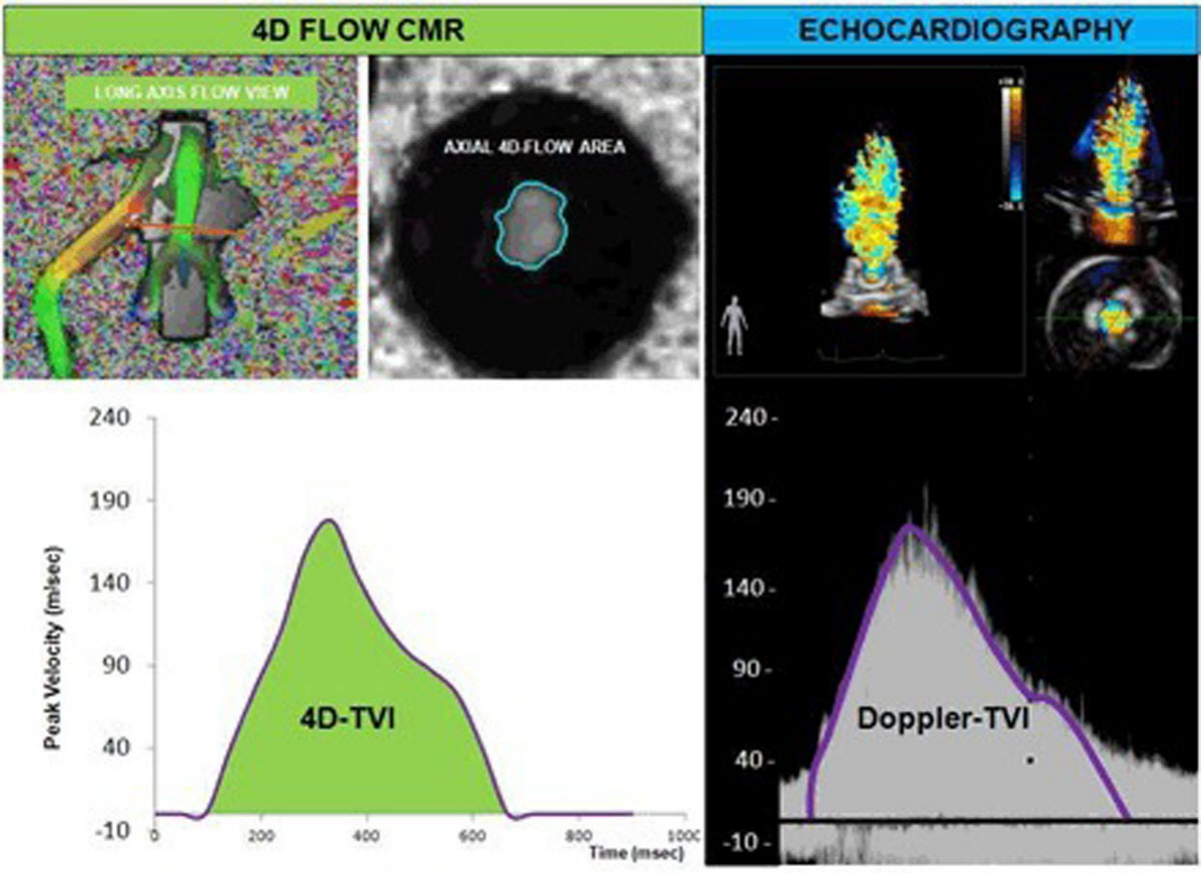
# Bioprosthetic mitral valve effective orifice area using 4D flow cardiac magnetic resonance derived time velocity integral. An in vitro comparison with Doppler Echocardiography
